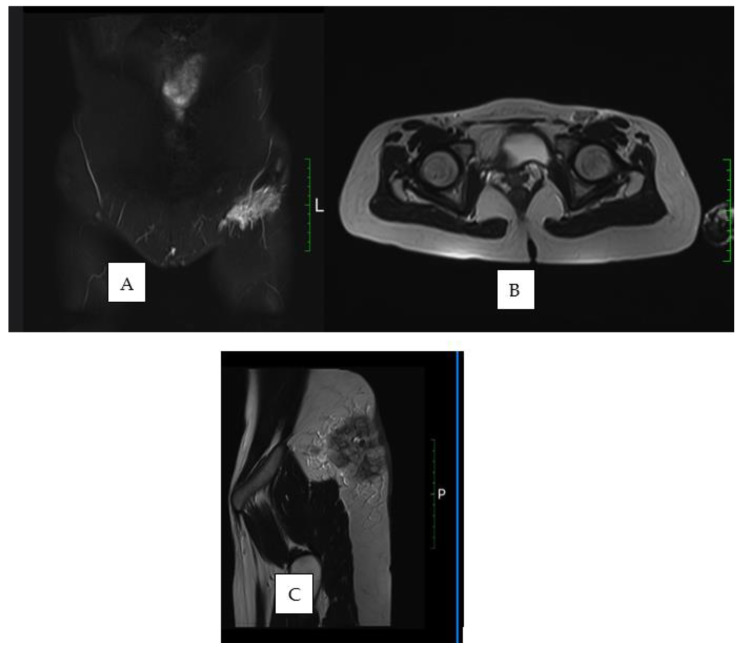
(A) Axial CT view showing left inguinal adenopathy; (B) Sagittal CT view revealing edematous and infiltrative changes in the left inguinal region; (C) Sagittal CT view of the gluteal region demonstrating a skin defect and profound subcutaneous edema.
